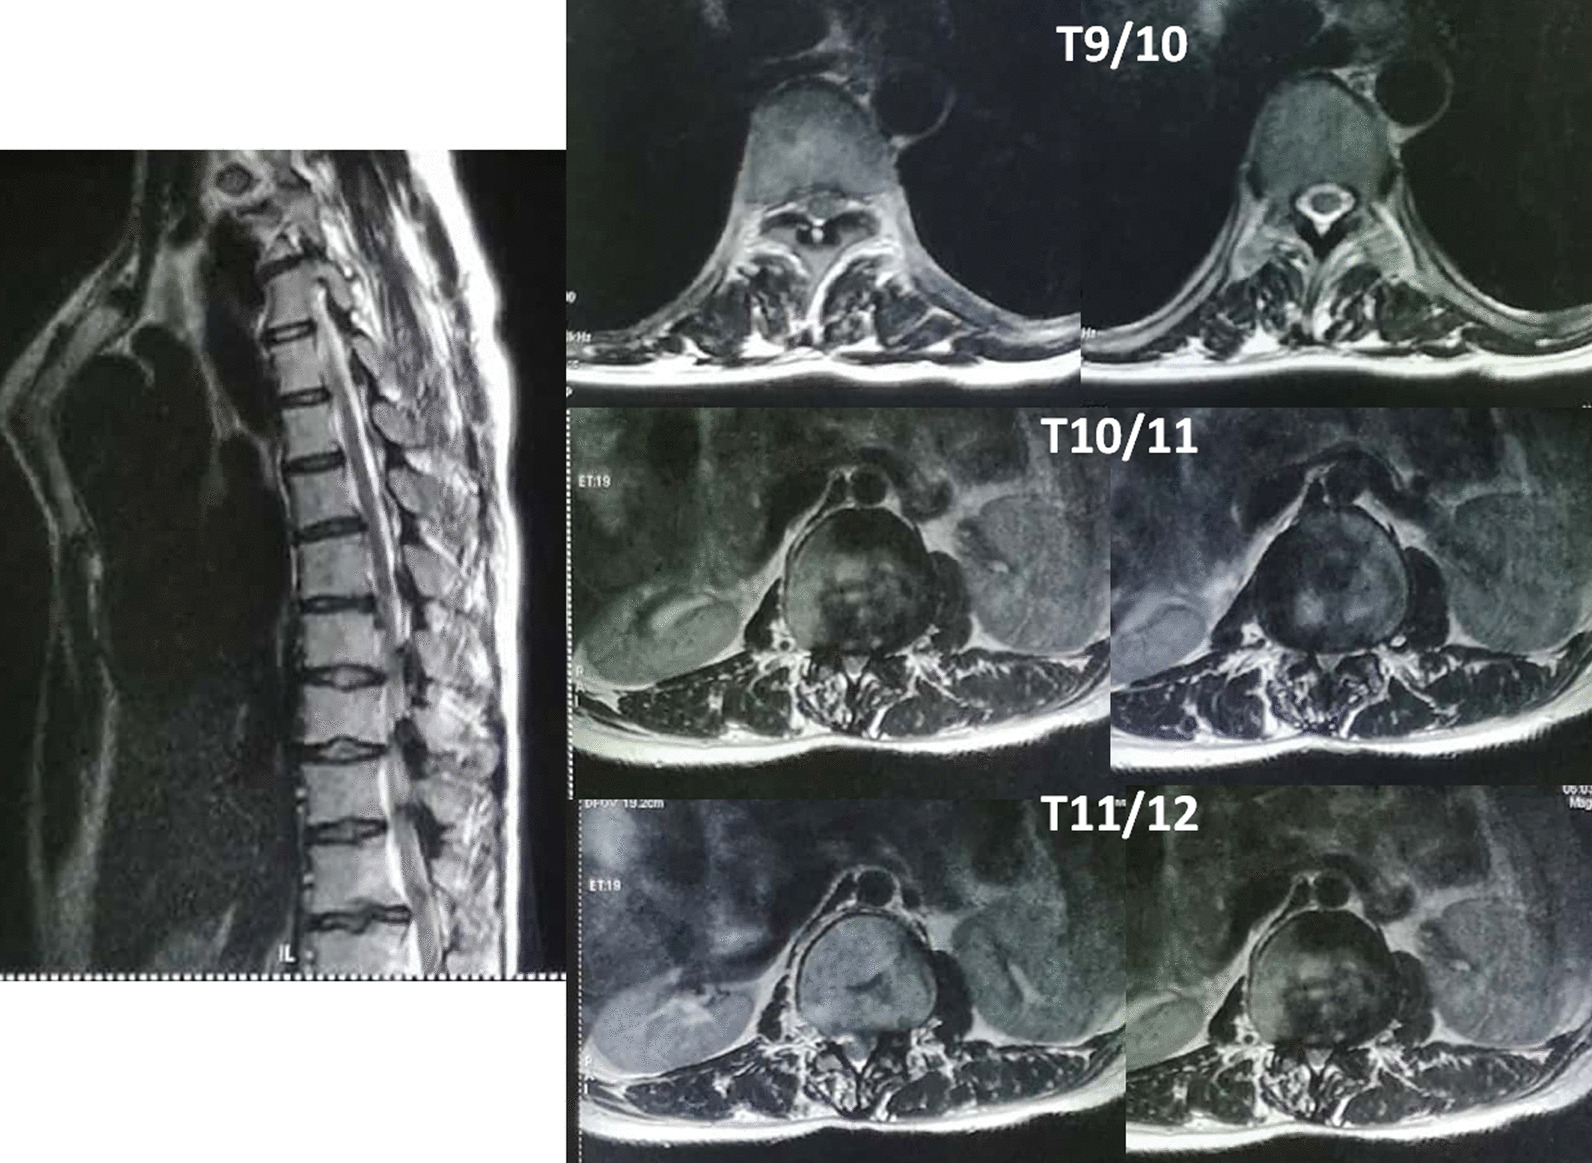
Sagittal and axial MRI of the thoracic spine showed multi-level severe thoracic spinal stenosis (T9/10, T10/11, T11/12) and spinal cord compression
